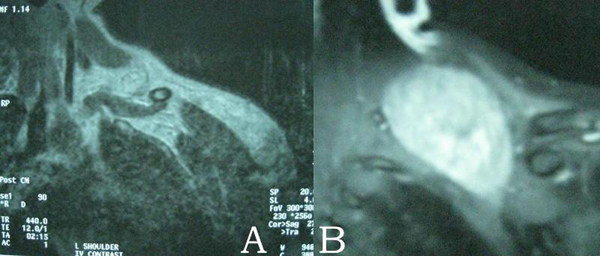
Magnetic resonance imaging of the neck shows on T1 (A) and T2 (B) sequencing a well-circumcised mass with increased signal intensity in the posterior cervical triangle, extending to the thoracic cavity.
